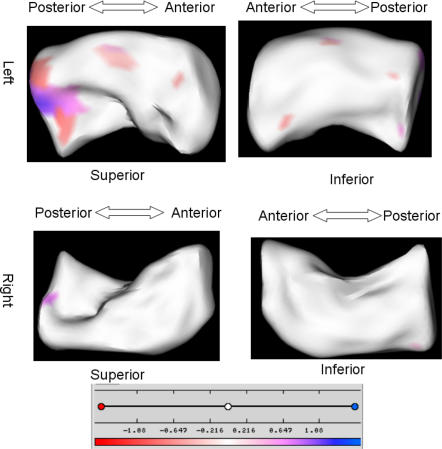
A pair of representative hippocampi are along with the T1 MRI scan from which the hippocampi were segmented.
